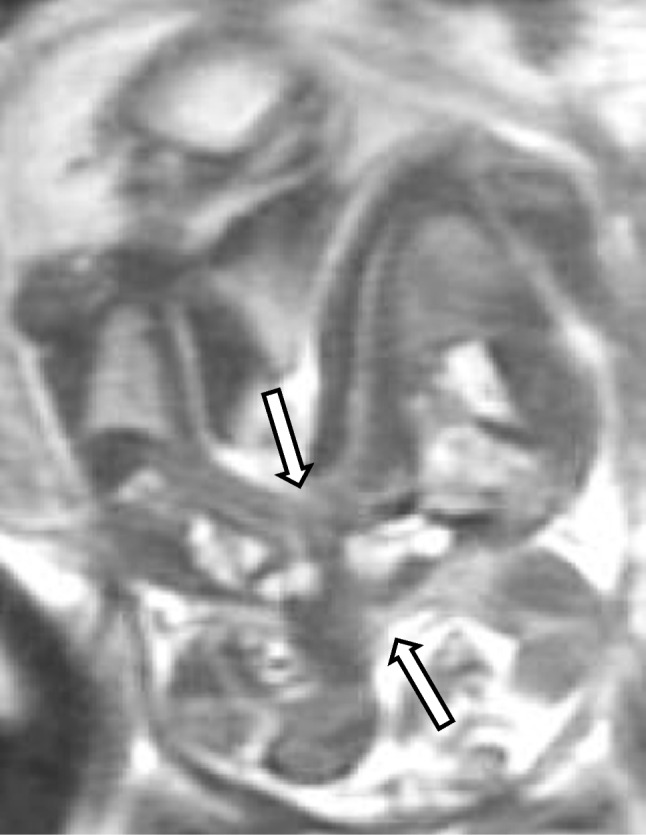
T2-weighted fetal MRI of pygopagus showing dorsal fusion in the sacrococcygeal region (*white arrows*). Image provided courtesy of Dorothy Bulas
